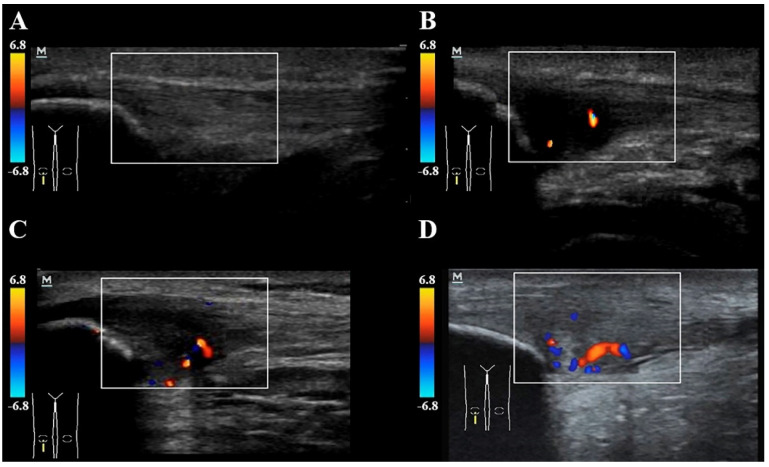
Intra-tendinous Doppler analysis for identifying neovascularization (A) grade 0: no visible vessels; (B) grade 1: 1 to 2 vessels within the ROI; (C) grade 2: 3 to 5 vessels within the ROI; (D) grade 3: vessels in up to 30% of the ROI. ROI: Region of Interest.
